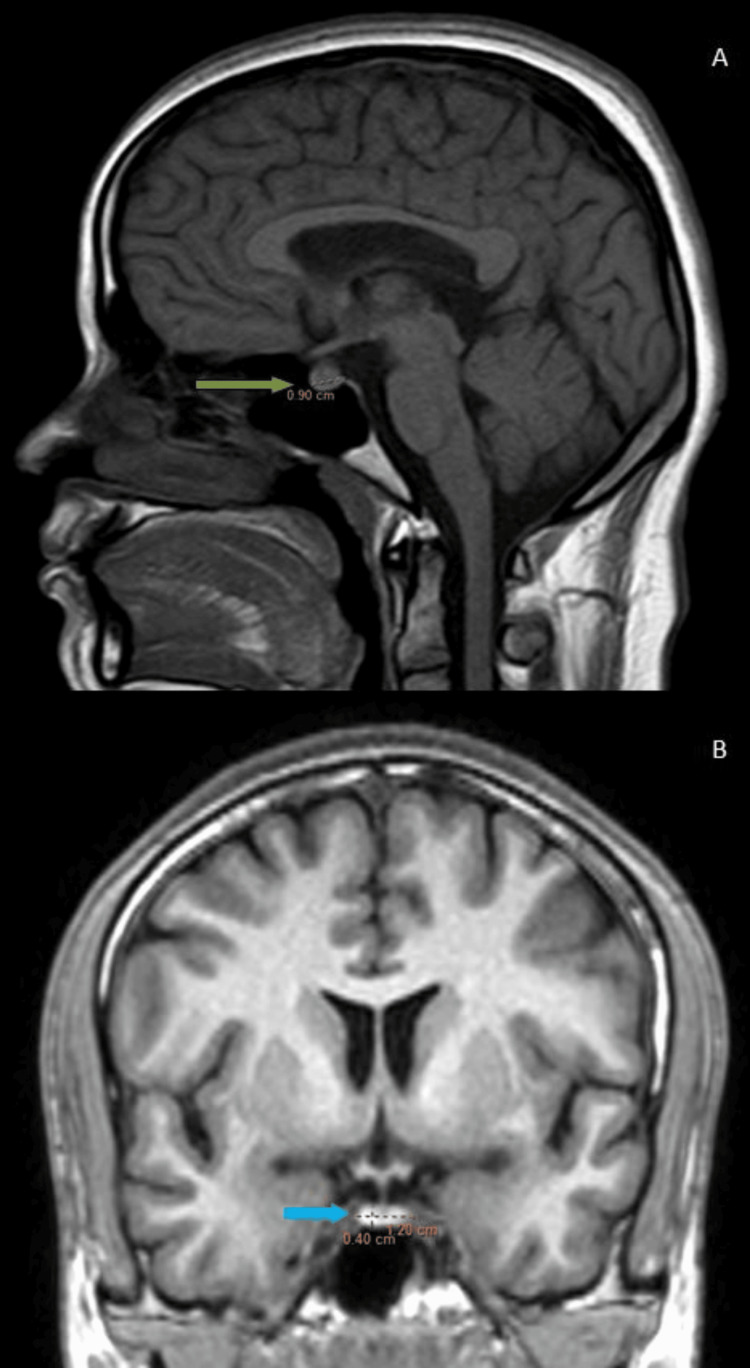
(A, B): MRI of the sella turcica. (A) T1 mid-sagittal: the pituitary gland in a 50-year-old male shows an anteroposterior of 9 mm (green arrow). (B) T1 mid-coronal: the pituitary gland of the same patient shows a transverse dimension and height of 12 mm and 5 mm, respectively (blue arrow). The pituitary gland volume is 270 mm3
